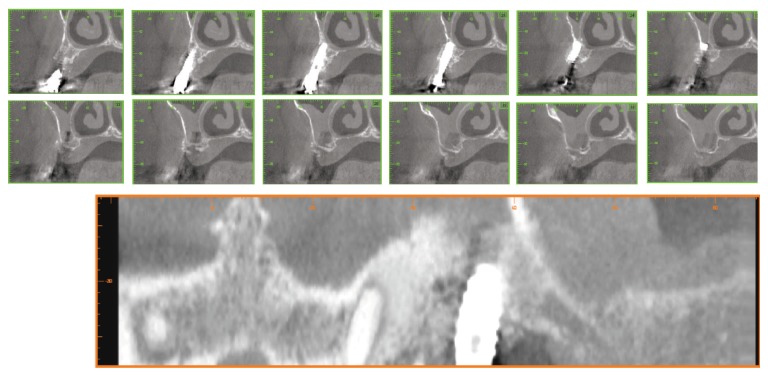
CBCT scans images after six months from surgical intervention showing a substantial stability of bone graft that allowed hypothesizing of the reosseointegration of the implant affected by peri-implantitis.
